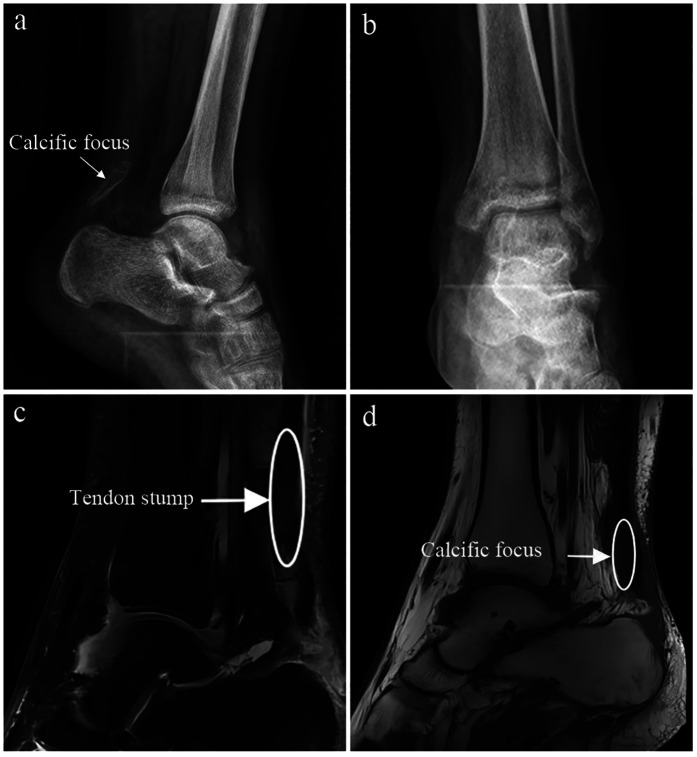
(a–d) Preoperative x-ray and magnetic resonance imaging (MRI) showing chronic rupture and defect of the left Achilles tendon with calcification.
